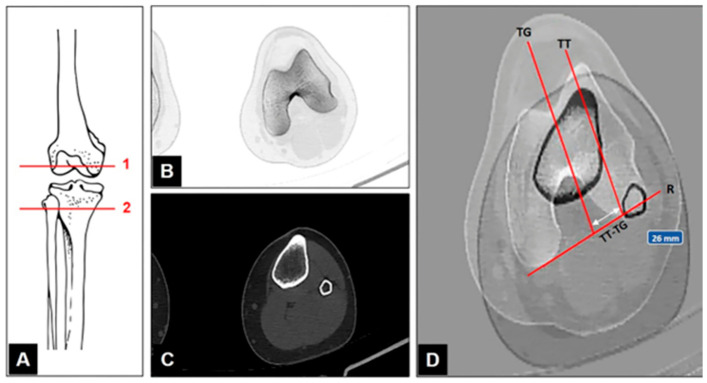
Analysis of the tibial tubercle–trochlear groove (TT–TG) distance using computed tomography: (A) selected slices 1 and 2; (B) slice corresponding to the deepest point of the femoral trochlea (slice 1); (C) slice of the apex of the tibial tubercle (slice 2); (D) superimposition of axial images and TT–TG assessment (slices 1 and 2). (R) posterior femoral transcondylar line; (TG) perpendicular line positioned at the deepest point of the femoral trochlea; (TT) perpendicular line positioned at the apex of the medial tibial tubercle.
